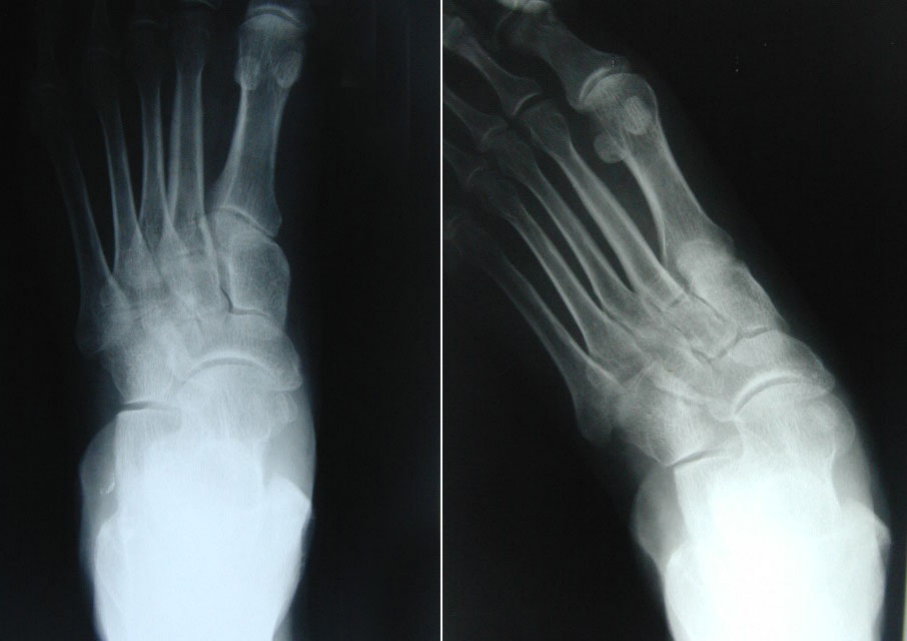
Anteroposterior X-ray of left foot. Right X-ray shows the standing anteroposterior view of the left foot with instability and subluxation of the talonavicular and the calcaneocuboid joints and on the left X-ray without weight bearing.
